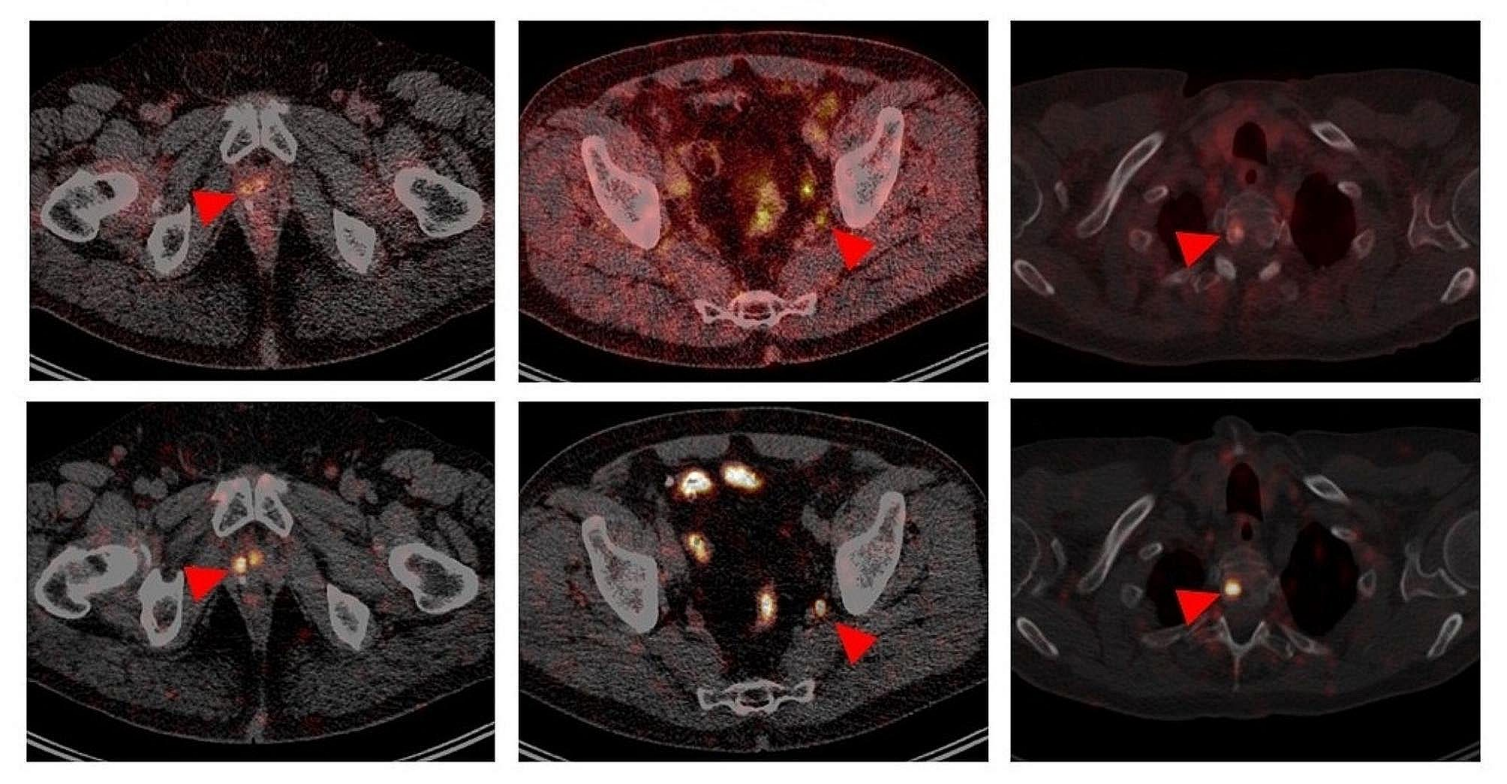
Representative transversal slice images, from 3 different patients (one per column), showing lesions (indicated by red arrows) that were indeterminate on [^68^Ga]Ga-PSMA-11 PET/CT (top row) but could be visually classified as suspicious (positive) for, respectively (bottom row, left to right), local recurrence, lymph node metastasis, and bone metastasis of prostate cancer on [^89^Zr]Zr-PSMA-617 PET/CT (48-hr scans shown here)
